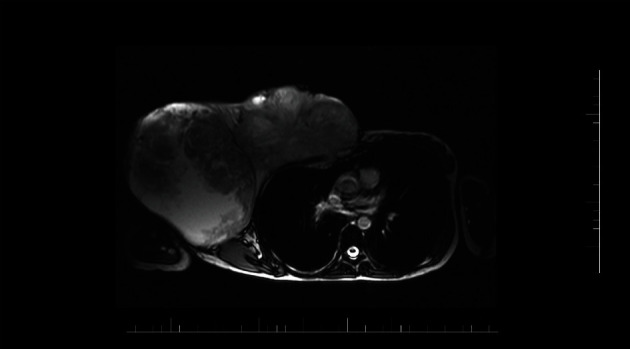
Magnetic resonance image (MRI T2 WI trufi tra) shows the overgrowth of the giant tumor into the thoracic wall at the level of the sternal ending of the third and fourth ribs with the thorax deformity. The MRI scan corresponds with the last recurrence of the tumor.
